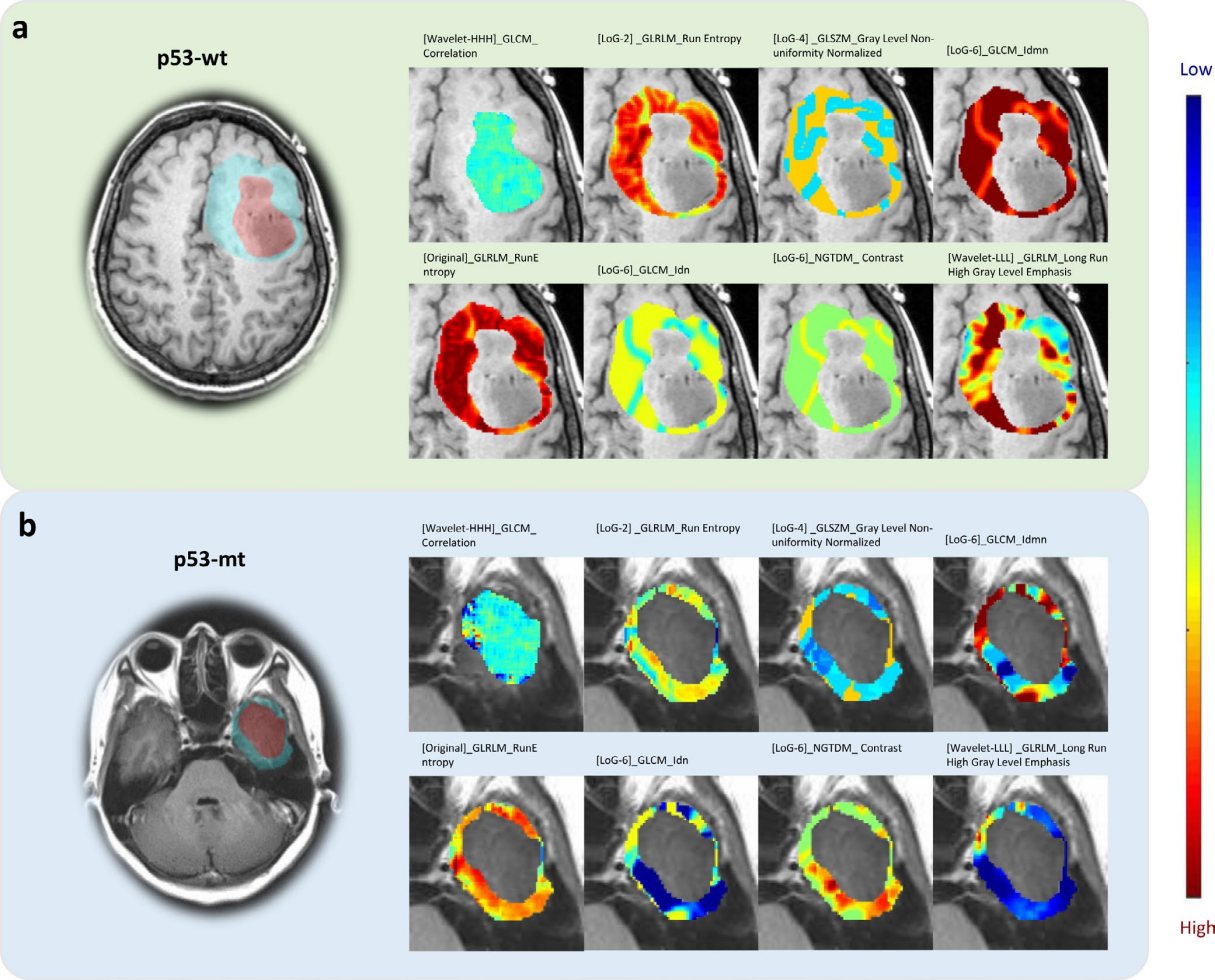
Texture feature maps for the selected radiomic signatures from VOI^T^ and VOI^PE^. (a) a 45‐year‐old woman diagnosed with p53‐wt in grade II glioma; (b) a 40‐year‐old woman diagnosed with p53 mutation in grade II glioma. Note that the MRI slice shown is the slice with the largest segmentation region. The segmented areas filled with red and blue represent the slice of VOI^T^ and VOI^PE^, respectively.
